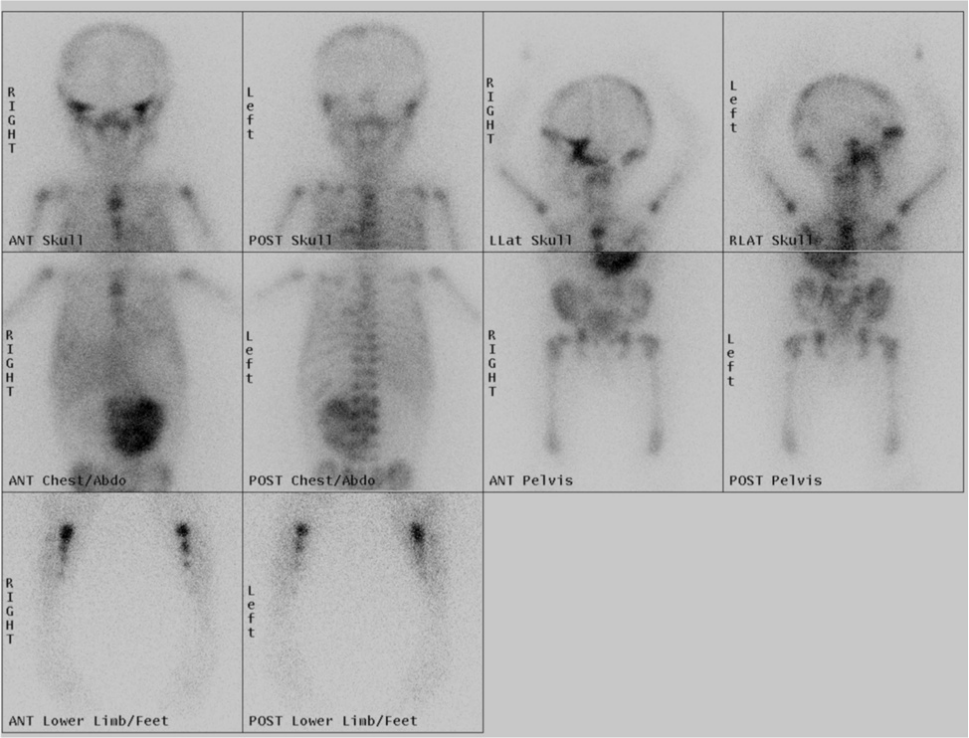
MIBG scintigraphy showing avid uptake at the site of the abdominal NBL with widespread bony metastatic spread.
